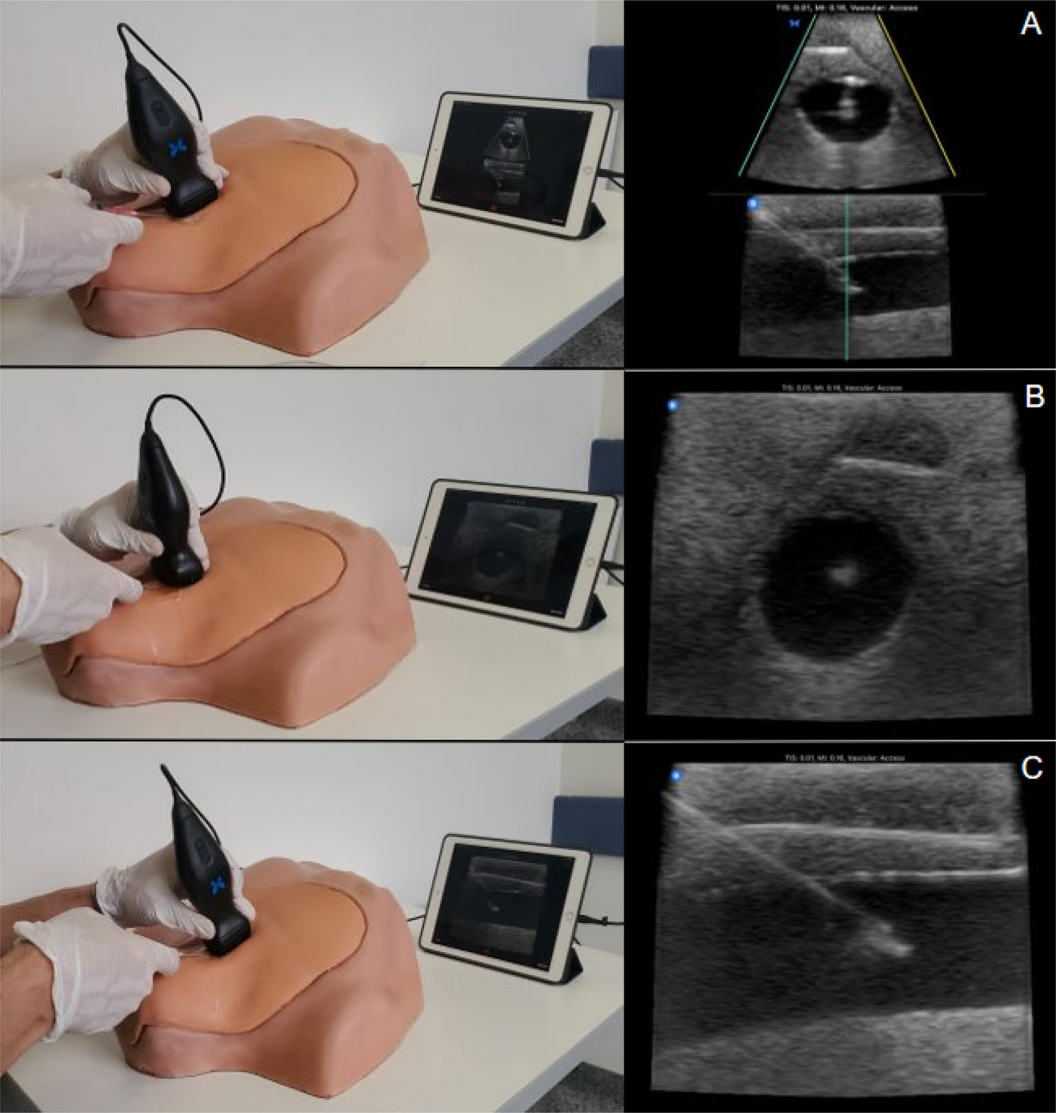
Ultrasound planes for vascular access. A Biplane, B Out-of-plane, C In-plane
